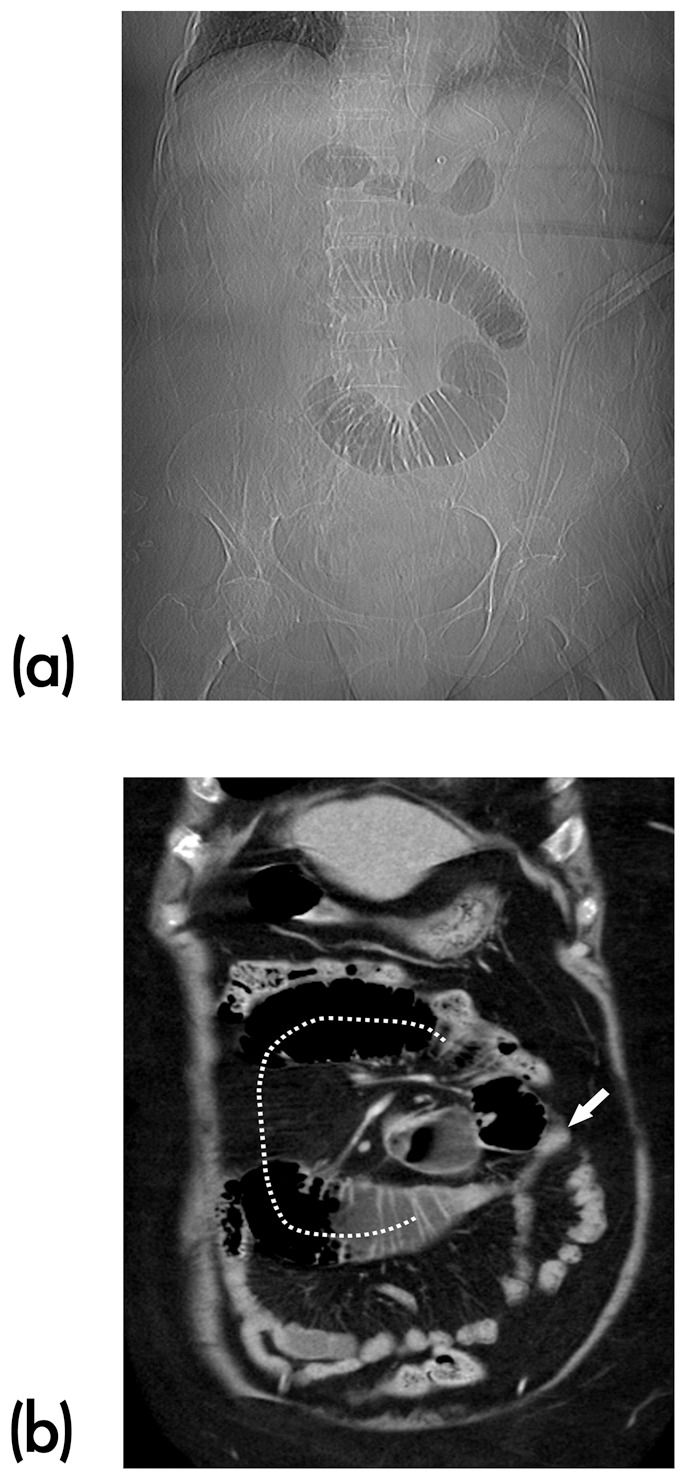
Closed-loop sign. (a) Abdominal radiograph shows a C-shaped configuration of the bowel loops in the center of the abdomen, a finding that indicates closed-loop obstruction. (b) On coronal MDCT image, the affected loops (dotted line) are filled with gas. The stretched mesenteric vessels converging toward the site of torsion (arrow).
